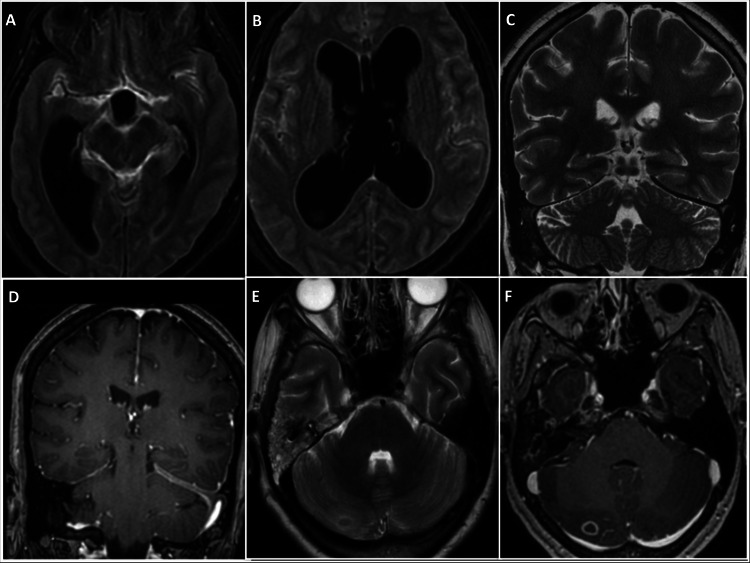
Imaging features in leptomeningitis, pachymeningitis, and tuberculoma (A, B) Leptomengitis with hydrocephalous: Post-contrast axial fluid-attenuated inversion recovery sequence (FLAIR) image demonstrates leptomeningitis affecting the bilateral Sylvian fissures, suprasellar cistern, and sulcal spaces, accompanied by dilation of the bilateral lateral and third ventricles. (C, D) Pachymeningitis : Coronal T2-weighted image shows hypointense thickening of the left-side tentorium (C). Coronal post-contrast T1-weighted image demonstrates dural enhancement along the left-side tentorium (D). (E, F) Tuberculoma: Axial T2-weighted image reveals a T2 hypointense lesion with mild perilesional edema (E). Post-contrast T1-weighted image demonstrates peripheral enhancement around the lesion (F).
